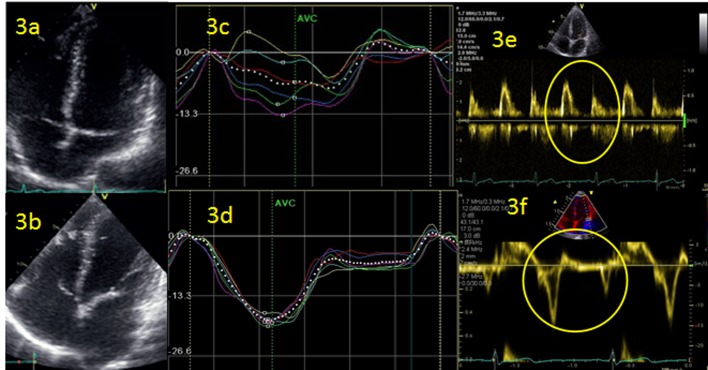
(a, b) Left atrium (LA) follow-up 2 years of triathlete with enlargement of LA and atrial fibrillation events. (b, c) Strain curves of left ventricle during atrial fibrillation (c) and 24 h after recovery in sinus rhythm (d). (e, f) Doppler measurement of diastolic function: conventional (e) and using tissue Doppler imaging (TDI).
